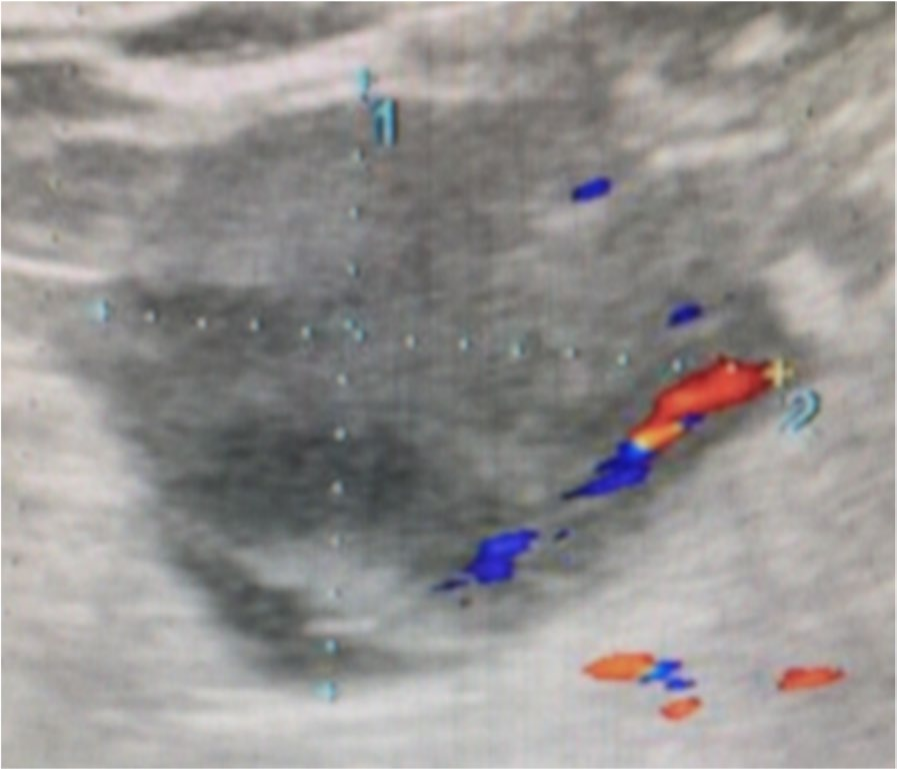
Ultrasound image of solid-cystic lesion
